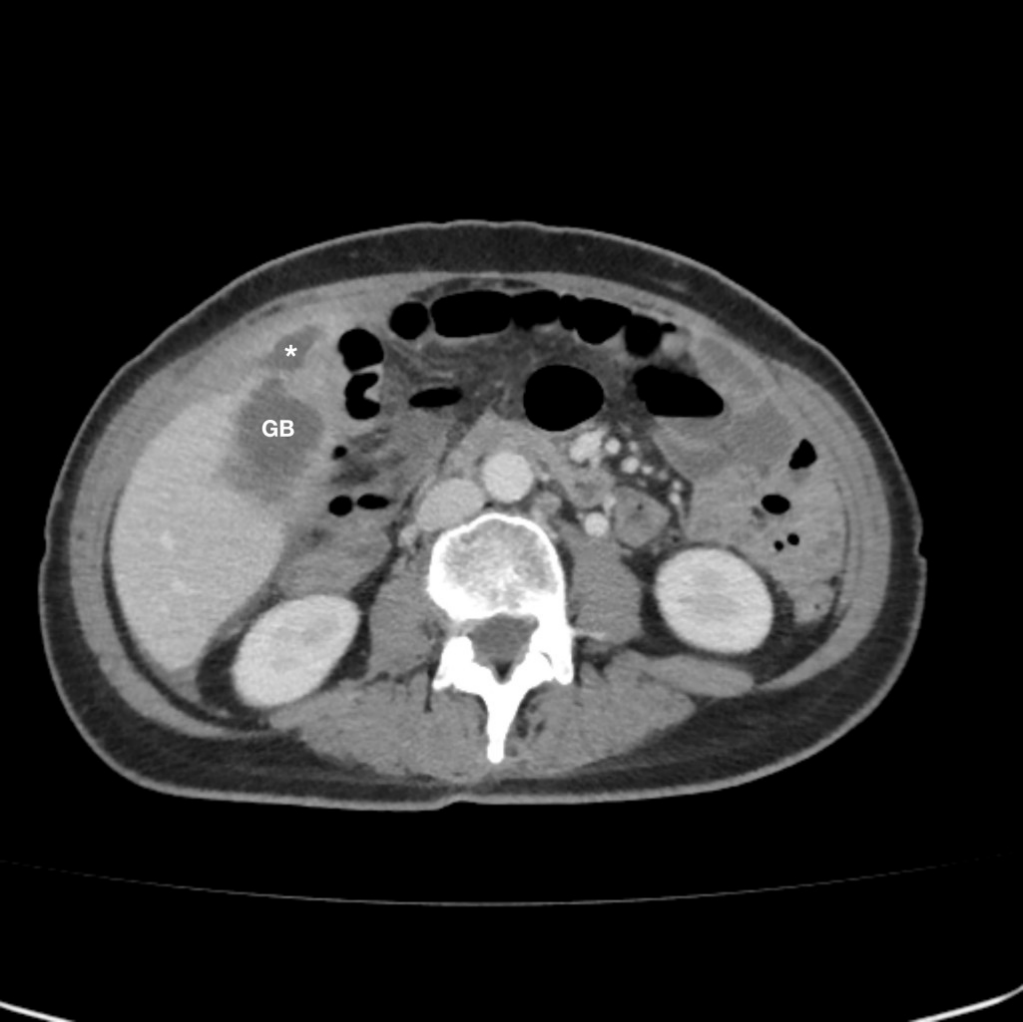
CT scan. The computed tomography (CT) scan shows a distended gallbladder with a thickened enhancing nodular wall and an adjacent fluid collection (*) concerning for gallbladder perforation.
